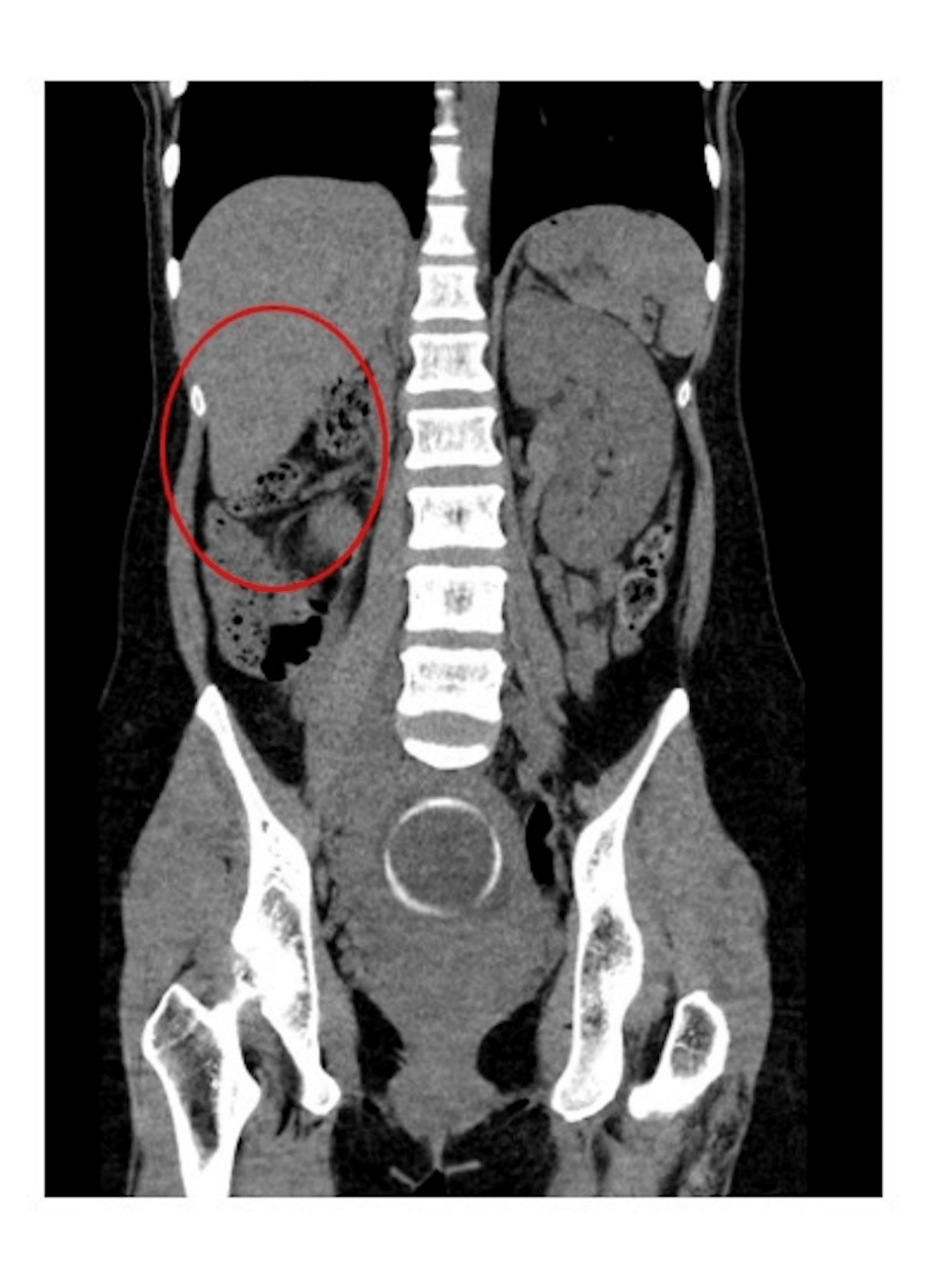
Computed tomography scan (coronal section) showing an absent right kidney (red circle).
